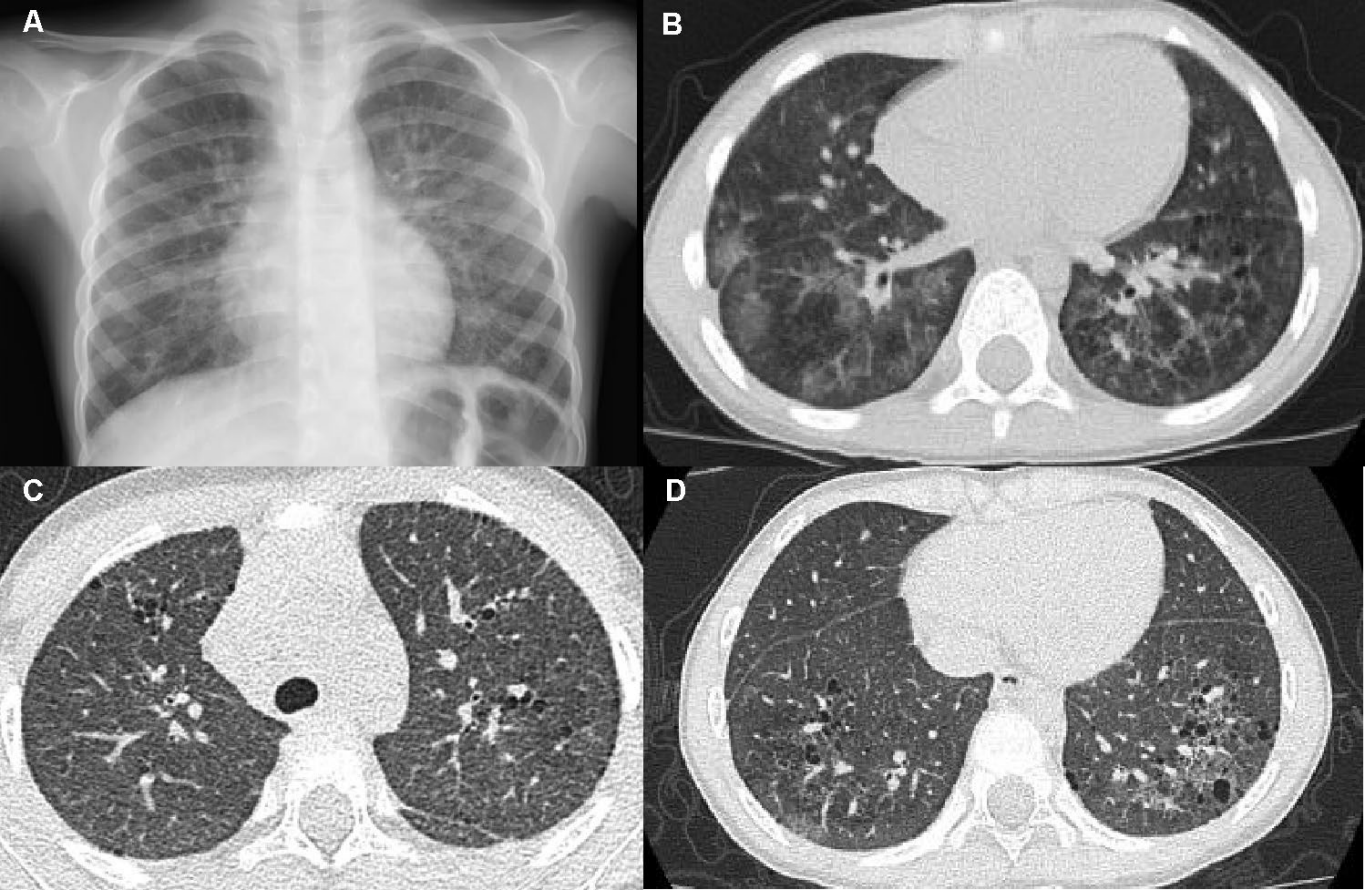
A mild manifestation of SP-C deficiency. A 5-year-old child with prolonged bloody sputum, triggered by viral respiratory infection. A CXR showed parahilar increased densities of both lungs. B CT displayed patchy GGOs intermingled with small cysts varying in size. C,D CT at age 8 years revealed clustering of much larger cystic lesions associated with mild GGOs in the central and peripheral zones of the lung
